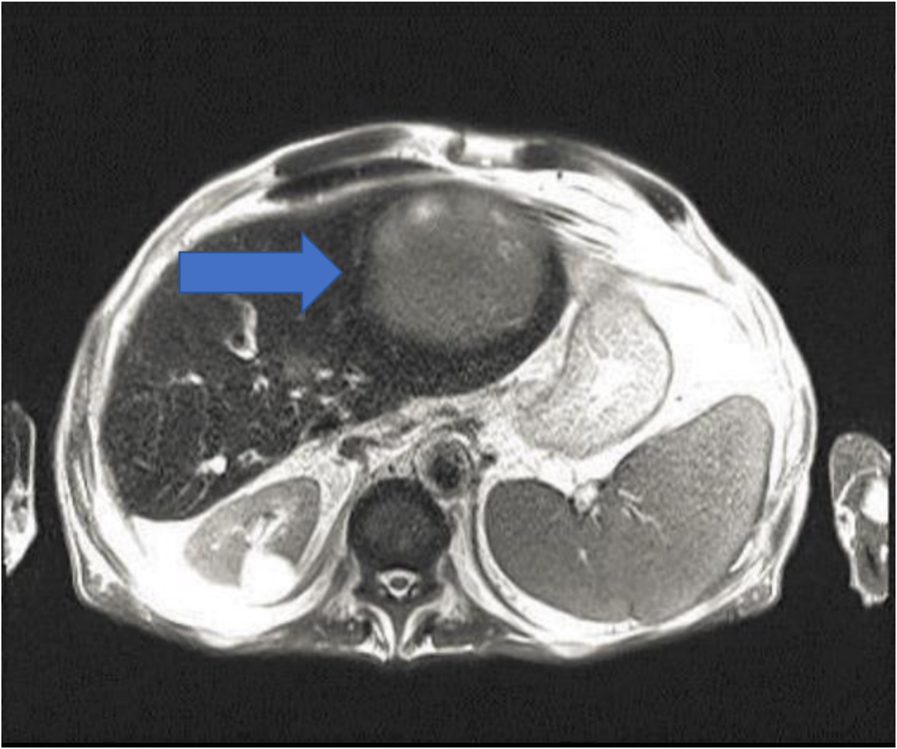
MRI-investigation 16 months after end of treatment. A rapid growth of the nodule in the left liver lobe was appreciable
